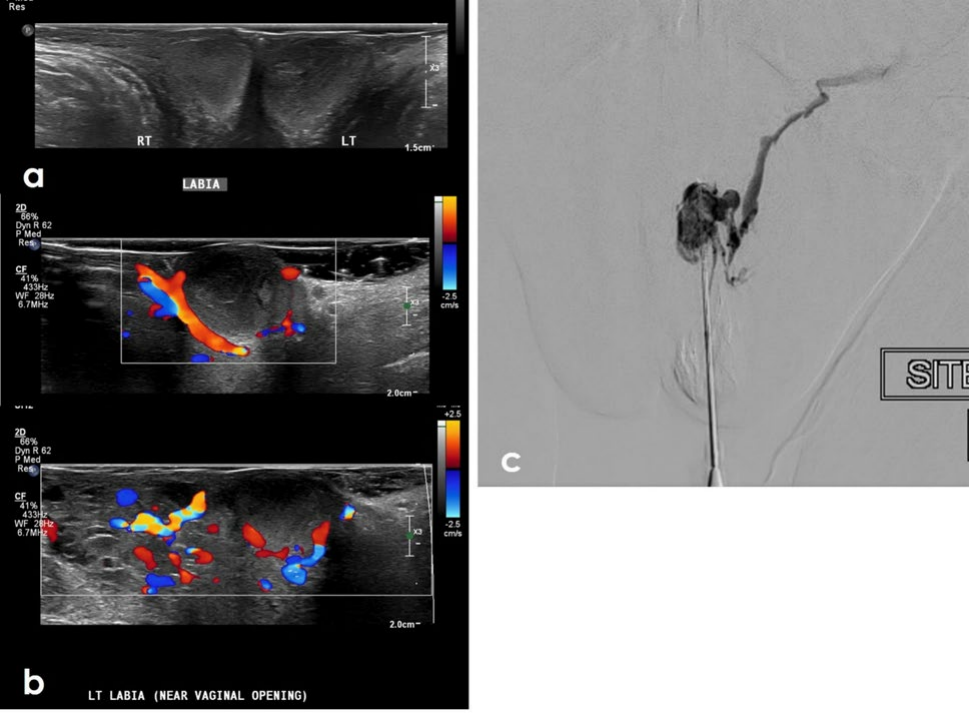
71-Year-old female with palpable labial mass. Grayscale (a) and color ultrasound (US) (b) images of the external genitalia show hypoechoic labial masses bilaterally. Tortuous vessels were noted in the adjacent soft tissues as well as the periphery of the lesions, which demonstrated slow flow internally on cine imaging (not shown). A diagnosis of labial varices was suggested. Embolization was performed by interventional radiology with resolution of the lesions and symptoms (c)
